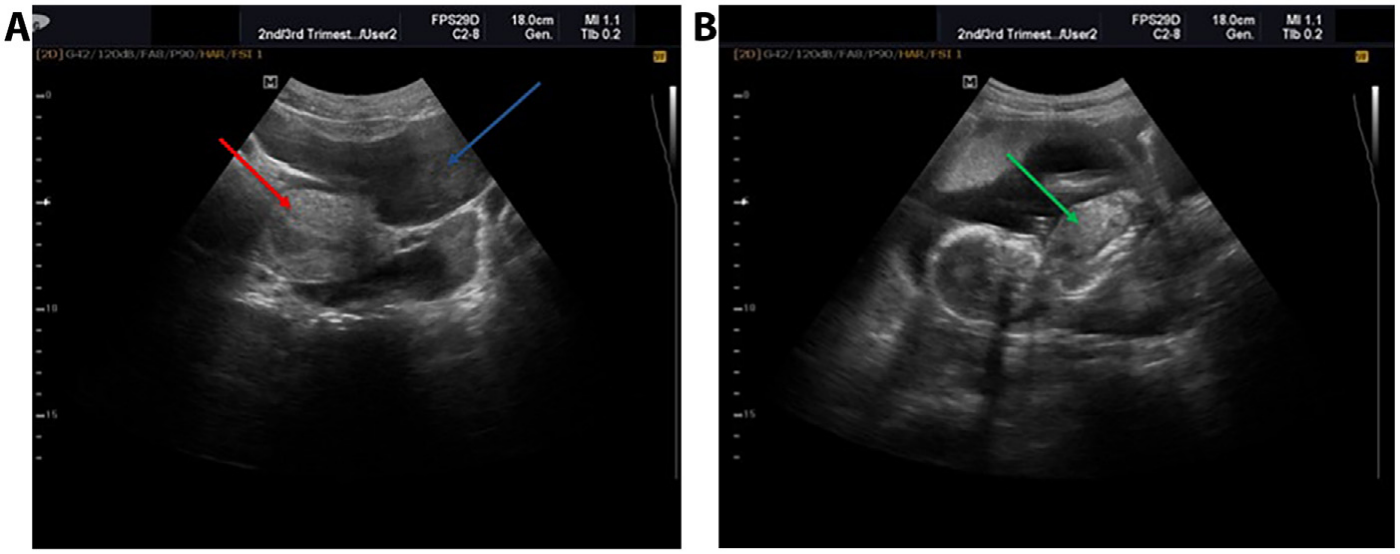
(A) Pelvic ultrasound showing an empty uterus with no intrauterine gestational sac (red arrow) and free peritoneal fluid extending toward Morrison’s pouch (blue arrow), contributing to the mistaken impression of an abdominal pregnancy. (B) Ultrasound image demonstrating a viable extrauterine fetus located outside the uterine cavity (green arrow), leading to an initial diagnosis of abdominal pregnancy before surgical confirmation of a ruptured rudimentary horn pregnancy.
